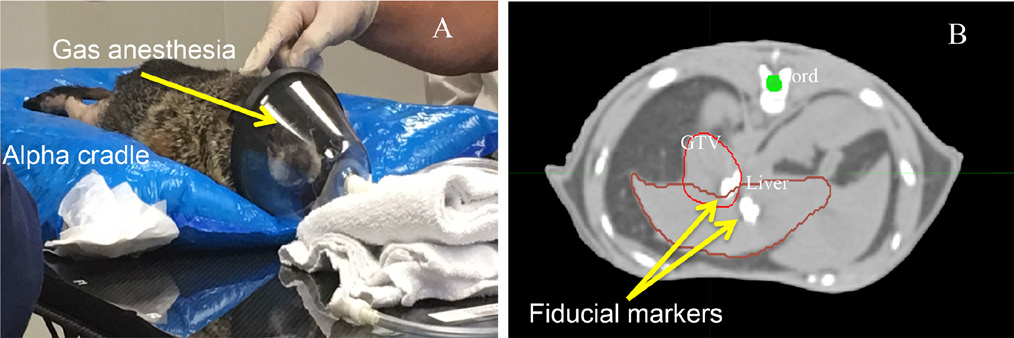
Experimental setup: (A) Woodchuck immobilized in the alpha-cradle mold. Gas anesthesia is administered throughout the treatment. (B) A CT slice to show the implanted fiducial markers and the contours outlined by the physician.
